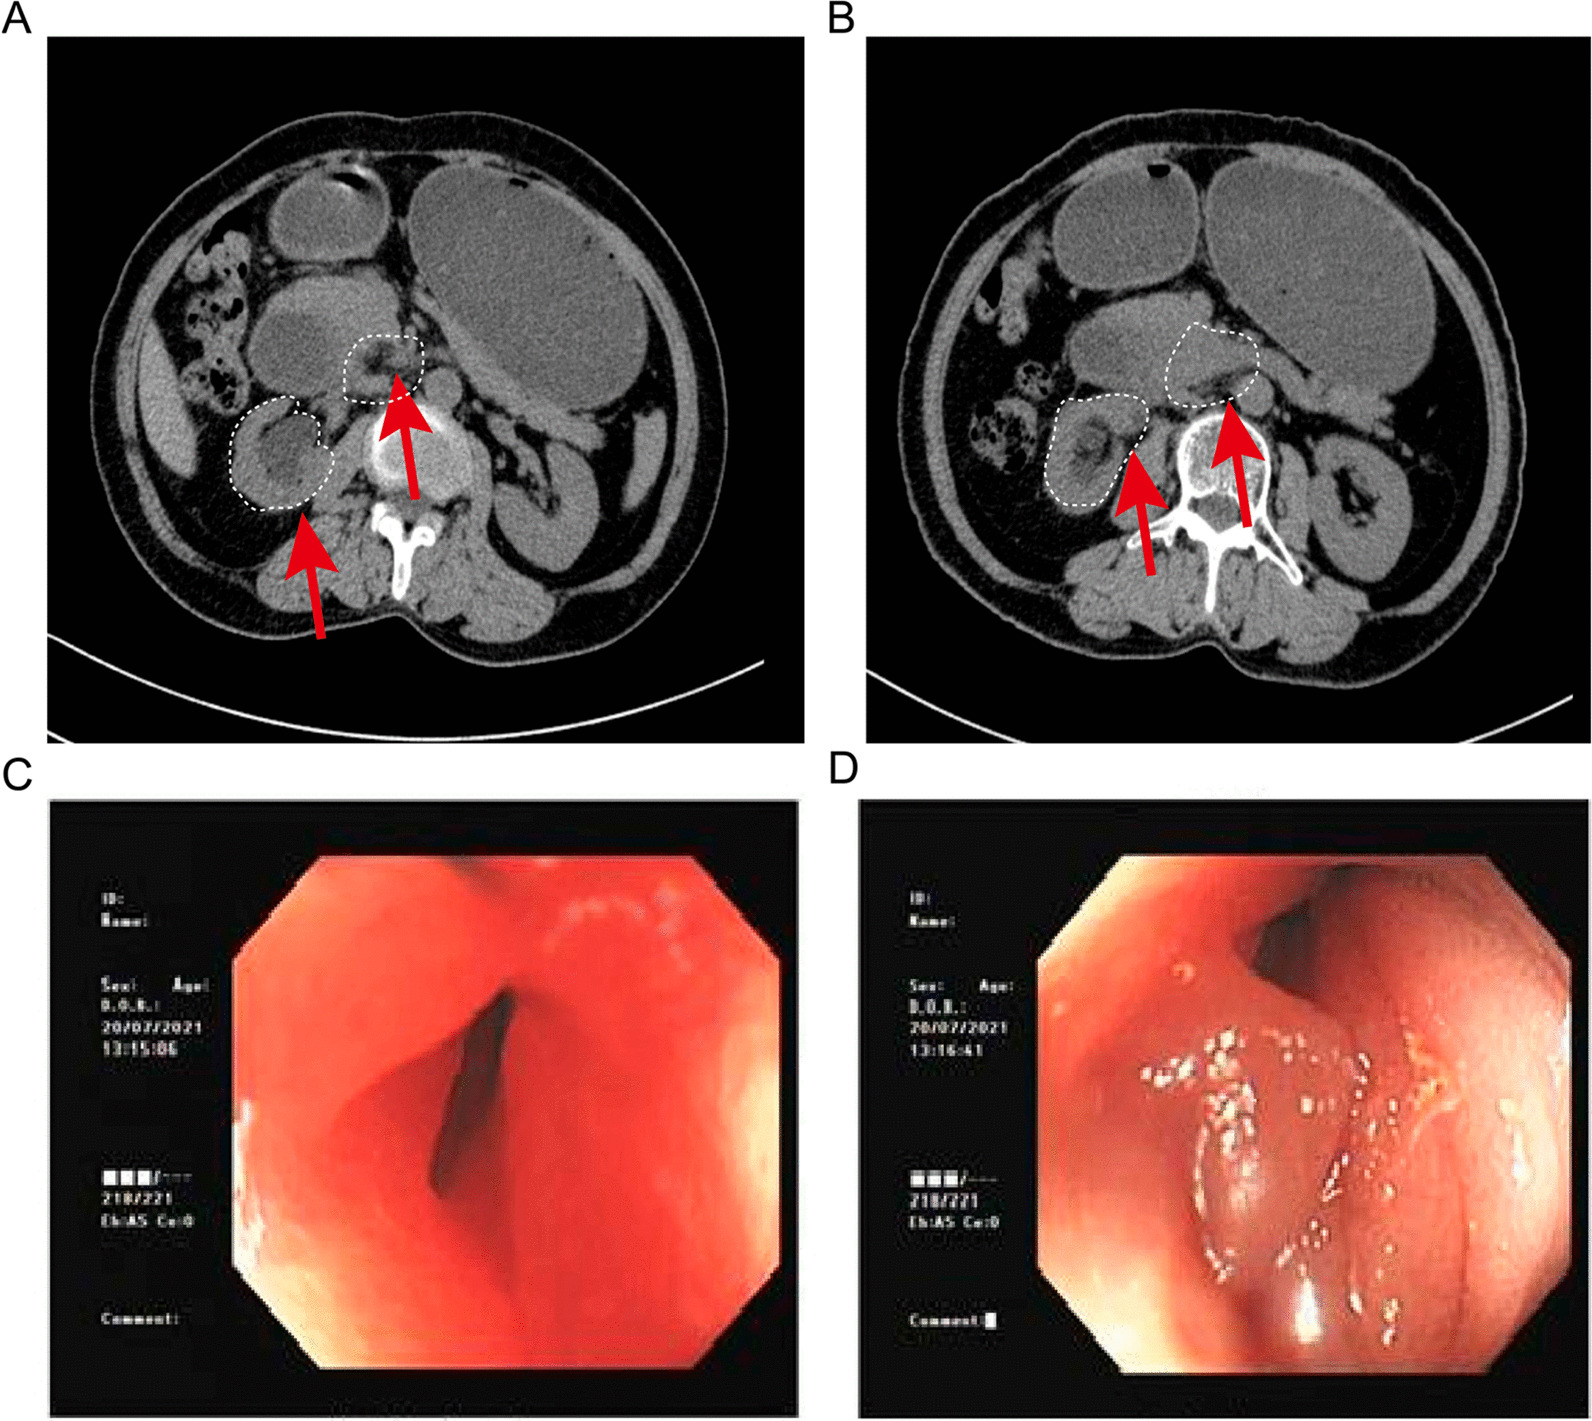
A and B, Computed tomography showed left hydronephrosis and thickening of the descending duodenum. C and D, Esophagogastroduodenoscopy (EGD) and small bowel endoscope revealed wall thickening and stenosis of the duodenum
